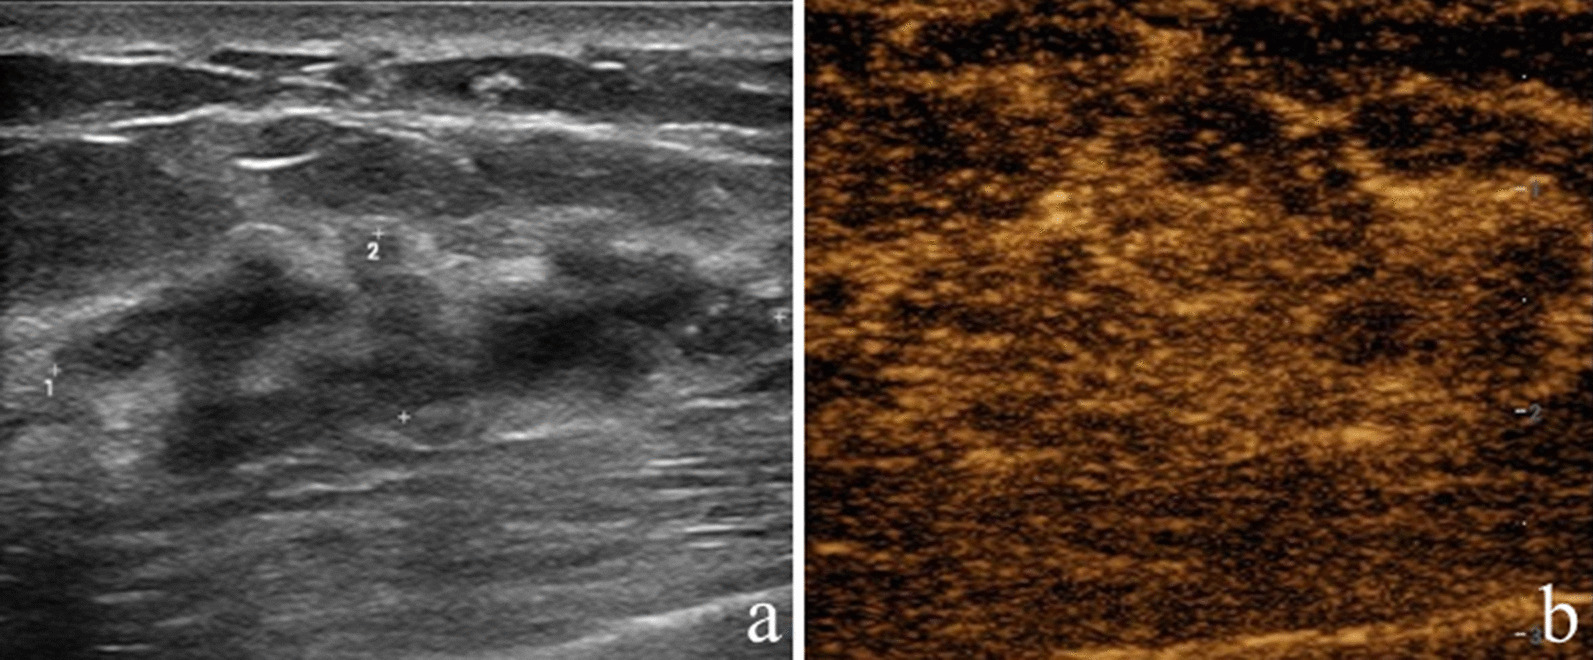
Image of a 60-year-old woman with a palpable mass. a B-mode ultrasound image; b contrast-enhanced ultrasound image showed that the lesion displayed iso-enhancement with the surrounding tissue. The CEUS score was 2 points. This lesion was originally classified as US BI-RADS 4B, downgraded to BI-RADS 4A after CEUS, and diagnosed as atypical ductal hyperplasia via US-guided core needle biopsy and as ductal carcinoma in situ at surgery
